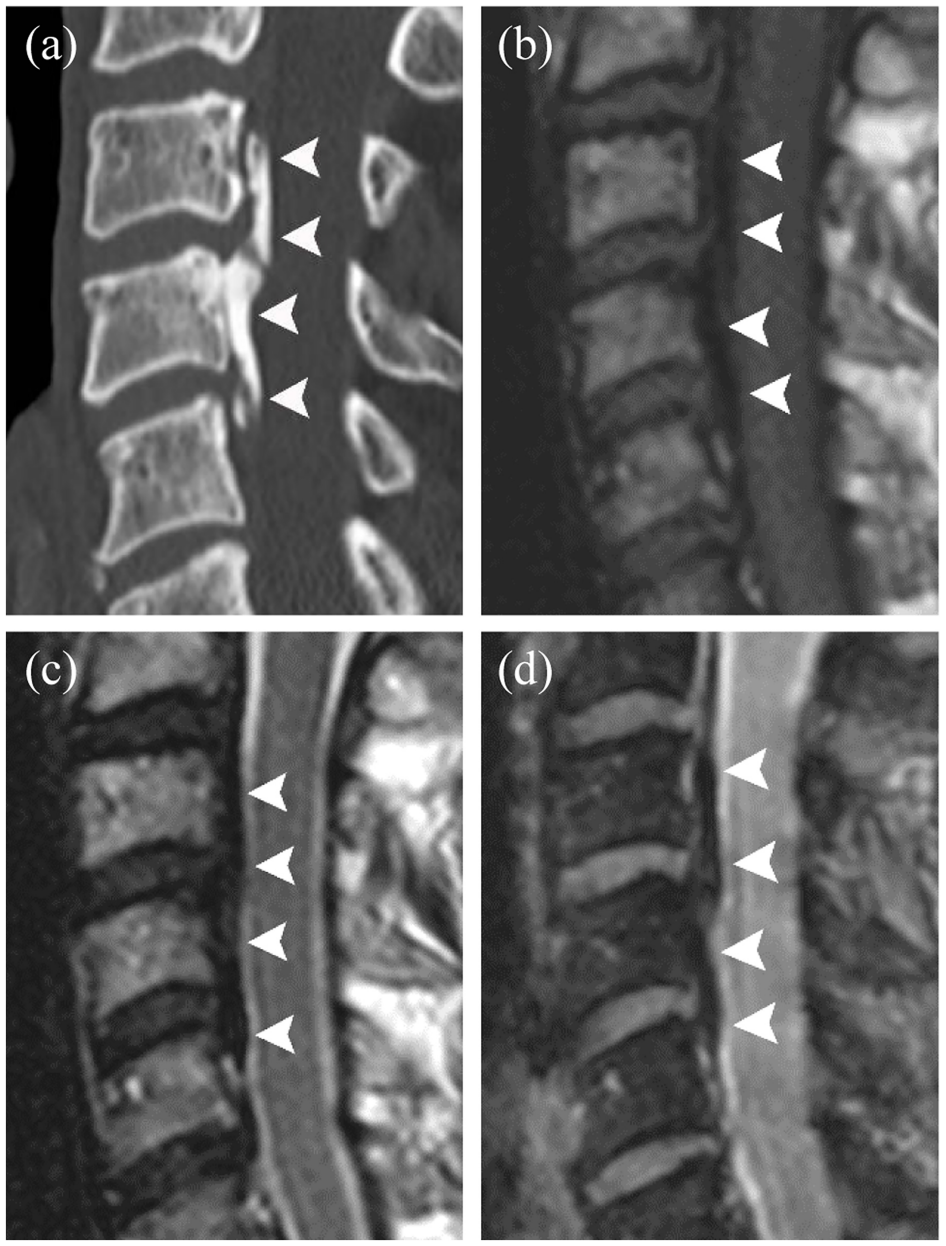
A 56-year-old man with cervical OPLL. (a) Sagittal CT of the cervical spine, showing continuous OPLL (*arrowheads*) at the level of the C3 through C5 bodies. (b) Sagittal T1WI, showing that the signal intensity of OPLL (*arrowheads*) is similar to that of the CSF. One reader interpreted these findings as an equivocal finding for OPLL, whereas another reader interpreted them as a probable absence of OPLL. (c) On the sagittal T2WI, the region of OPLL (*arrowheads*) is not clearly delineated, since its signal intensity is similar to that of the disc. Both readers interpreted these findings as an equivocal finding for OPLL (score 2). (d) mFFE image clearly showing a region of OPLL (*arrowheads*) exhibiting good contrast with the CSF and disc. This image was correctly interpreted as indicative of the presence of OPLL (score 4) by both readers.
